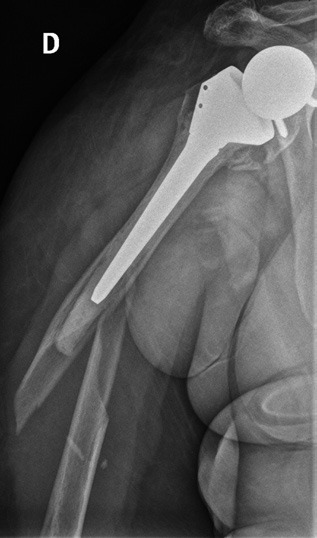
Front radiography showing reverse shoulder periarthroplasty fracture
